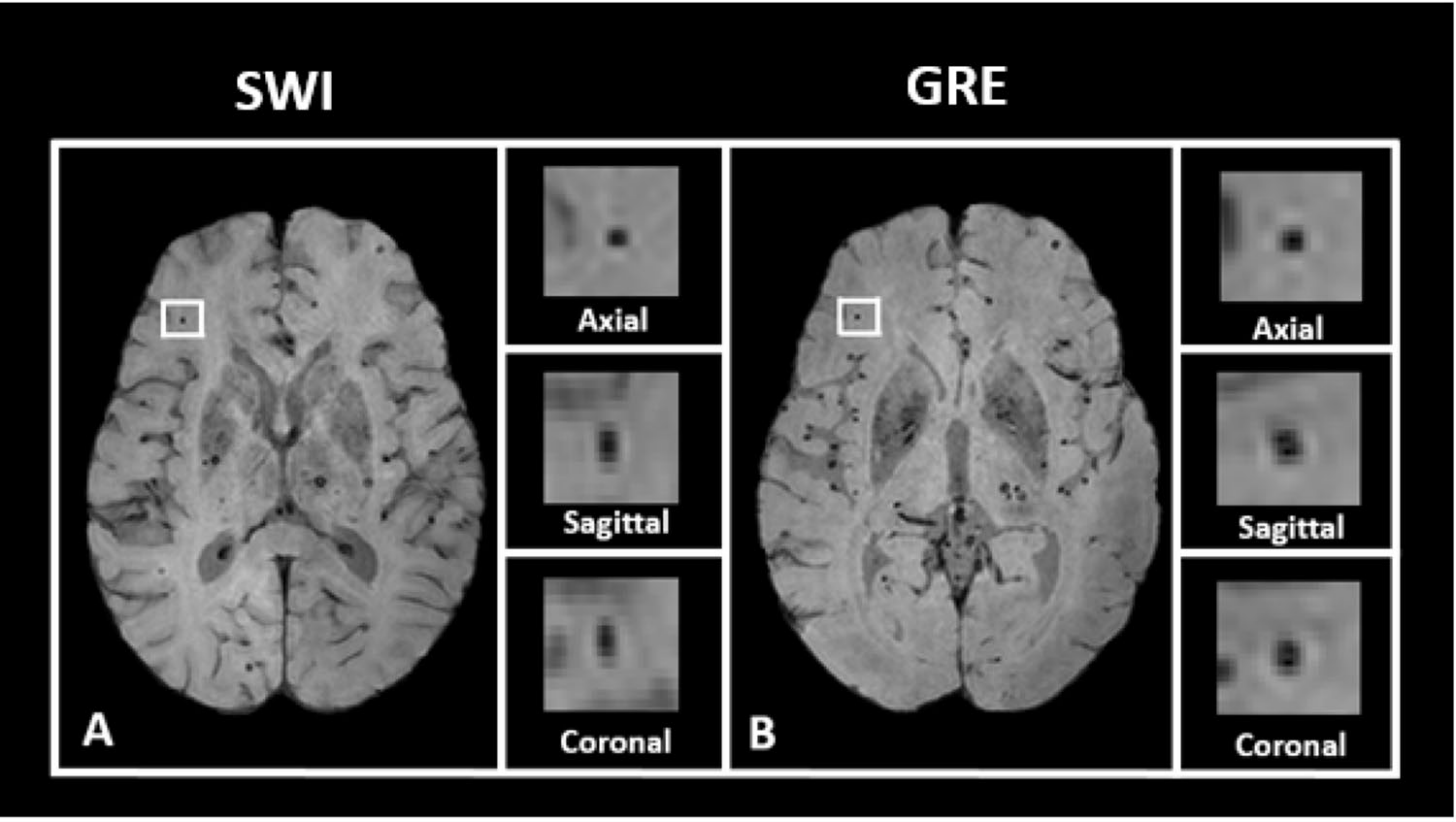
Examples of microbleed location. The participant’s MRI shown here reflects a heavy burden of cerebrovascular disease. (A) Left: axial slice of a SWI image, with a microbleed location highlighted in white. Right: an enlarged image of the highlighted location. From top to bottom, the views shown are axial, sagittal, and coronal. (B) Left: axial slice of the same participant’s GRE image, with the same microbleed location highlighted in white. Right: an enlarged image of the highlighted location. From top to bottom, the views shown are axial, sagittal, and coronal.
